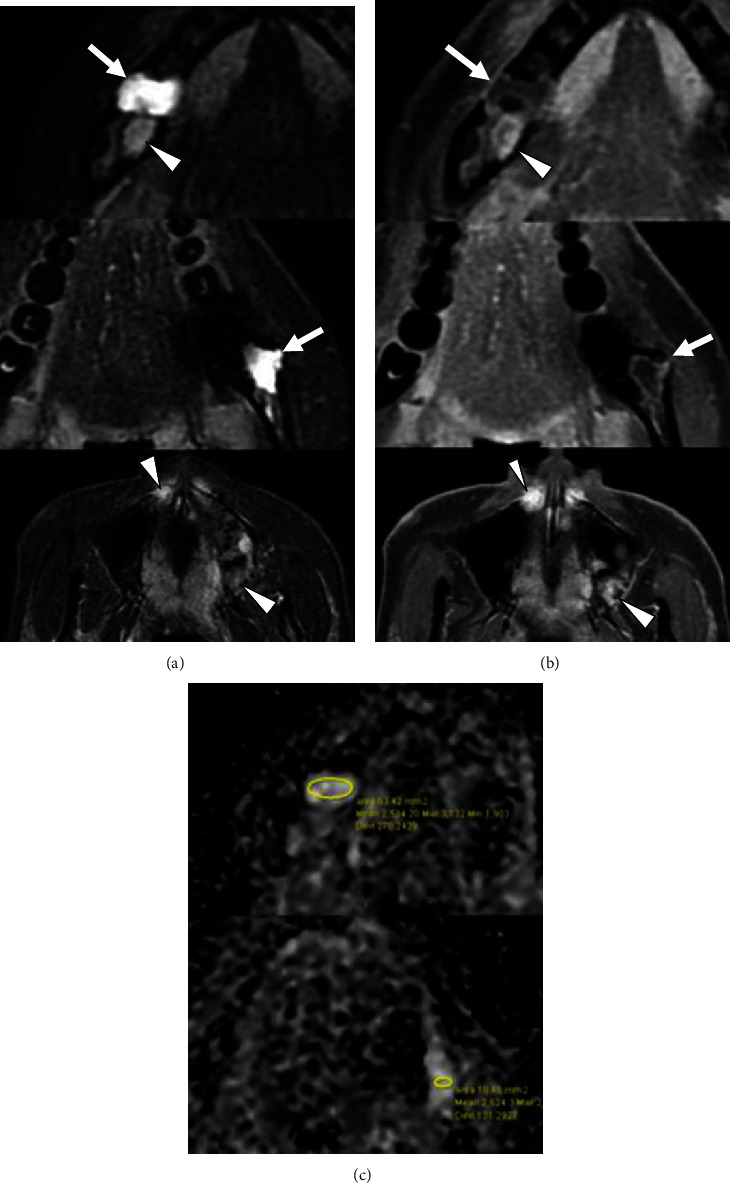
Magnetic resonance images. (a) Transverse fat-suppressed T2-weighted images show extremely hyperintense signal areas in the bilateral mandible (*arrows*) and hyperintense signal areas in the right mandible and bilateral maxilla (*arrowheads*). (b) Contrast-enhanced T1-weighted images show thin, peripherally enhanced lesions in the bilateral mandible (*arrows*) and heterogeneously enhanced lesions in the right mandible and bilateral maxilla (*arrowheads*). (c) The ADC maps show extremely high ADC values (over 2.5 × 10^−3^ mm^2^/s) at cystic areas in the bilateral mandible. ADC: apparent diffusion coefficient.
